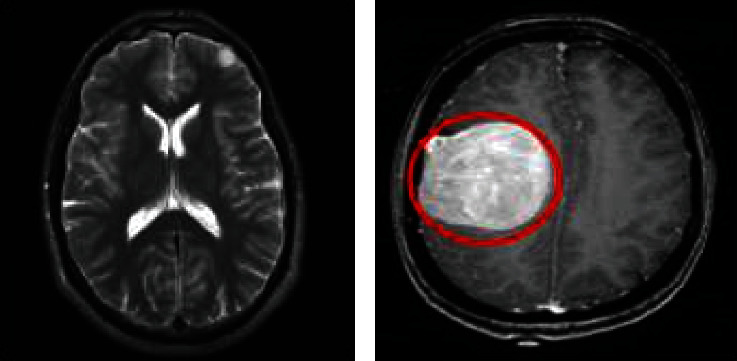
Visual insights of brain MRI images: (a) normal brain and (b) tumorous brain.
